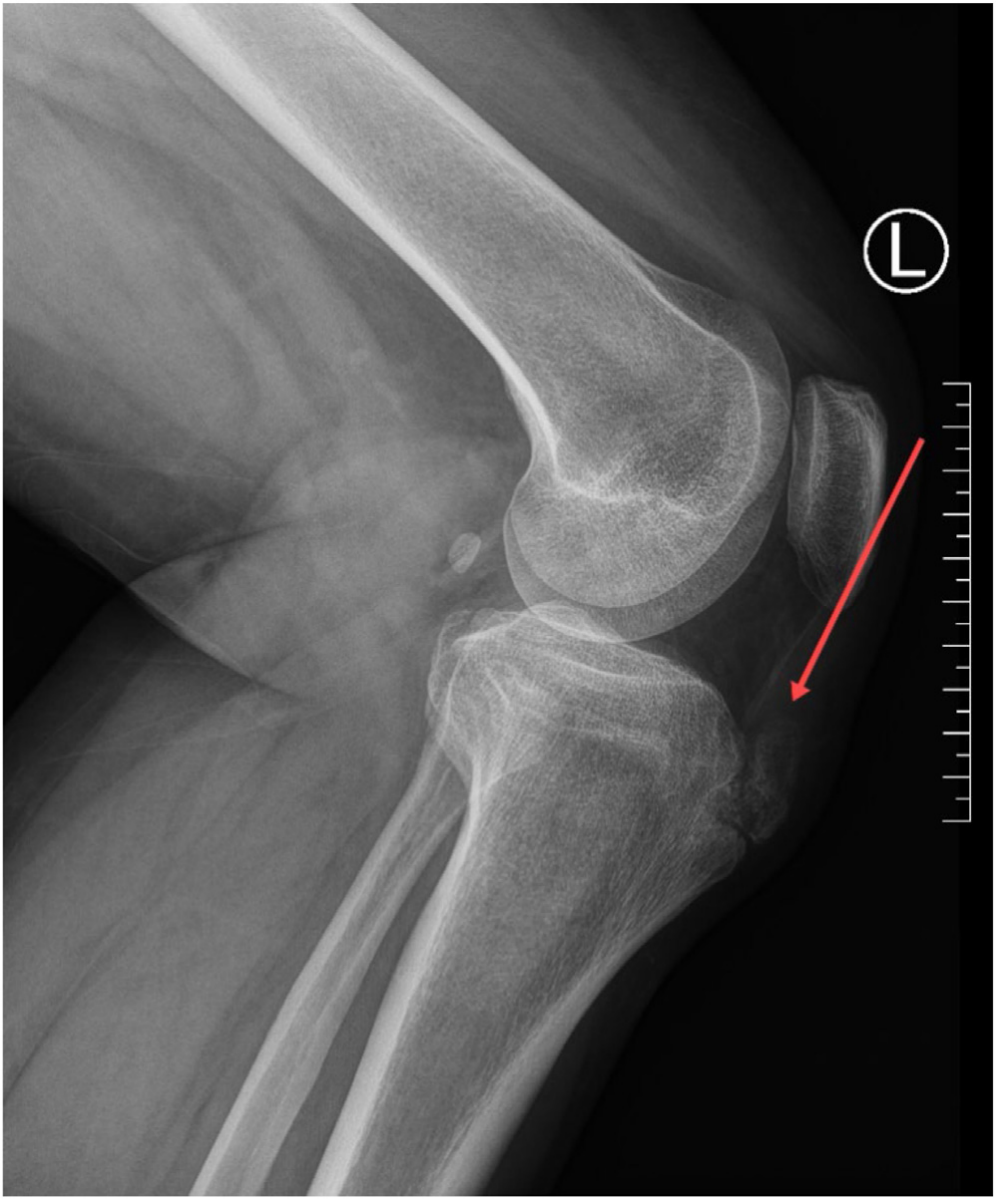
The sagittal view of the x-ray of the patient’s left knee. The red arrow points to the tibial tubercle osteophyte, consistent with the characteristics of Osgood-Schlatter disease.
